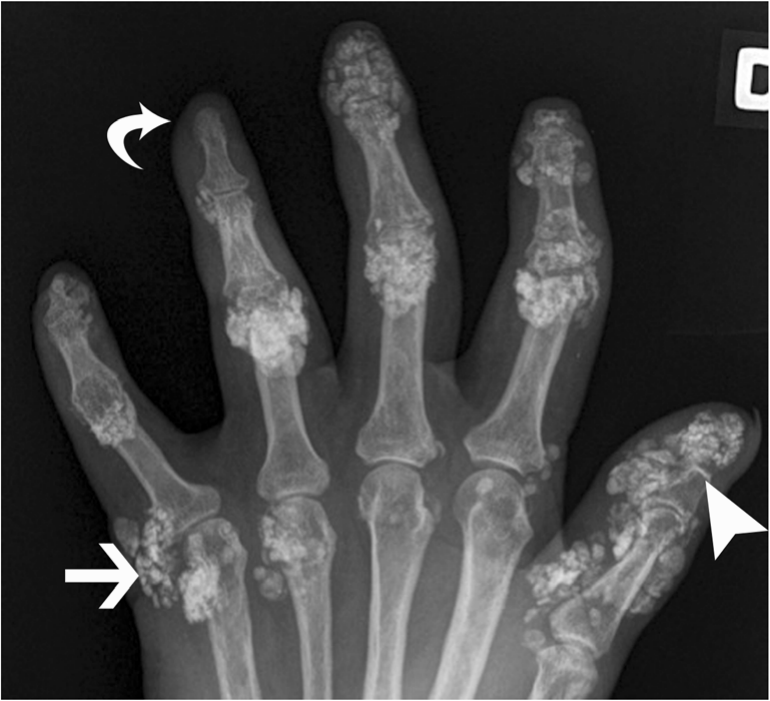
Systemic Sclerosis. Posteroanterior radiograph of the hand shows tumoural calcinosis in the soft tissue (arrow). Note acro-osteolysis (arrowhead) and atrophy of soft tissue (curved arrow), characteristic of systemic sclerosis
